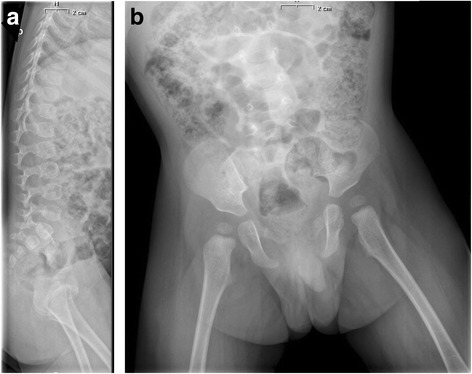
in figure the spine and pelvis X-ray in laterocervical a and in anteroposterior b projections is represented
